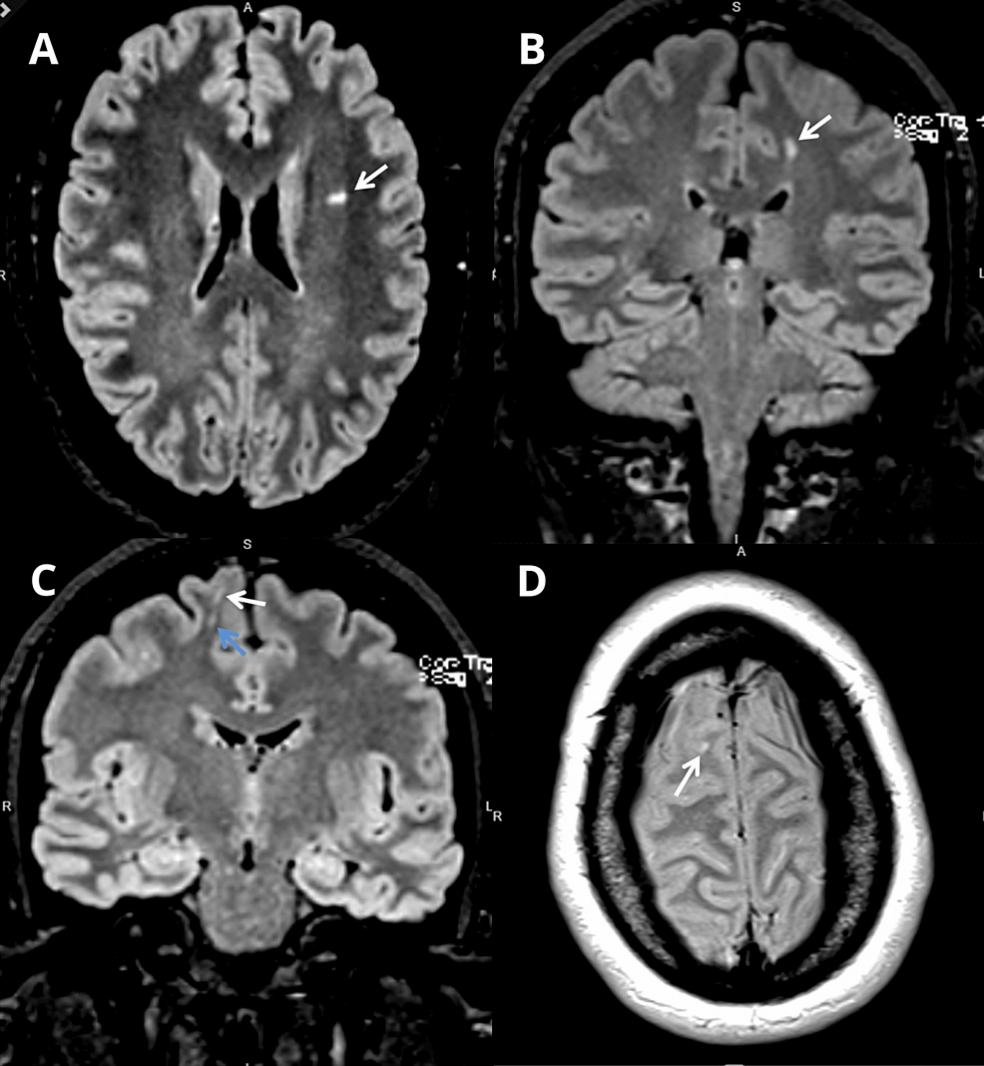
Brain MRI revealing areas of focal hypersignal in T2 (A) Brain MRI, axial T2 FLAIR, hyperintense signal in the anterior region of the left corona radiata (arrow). (B) Brain MRI, coronal T2 FLAIR, hyperintense signal in the left parasagittal frontal subcortical region (arrow). (C) Brain MRI, coronal T2 FLAIR, hyperintense signal in the right juxtacortical (white arrow) and superior frontal subcortical (blue arrow) regions. (D) Brain MRI, axial T2, hyperintense signal in the right juxtacortical region (arrow).
